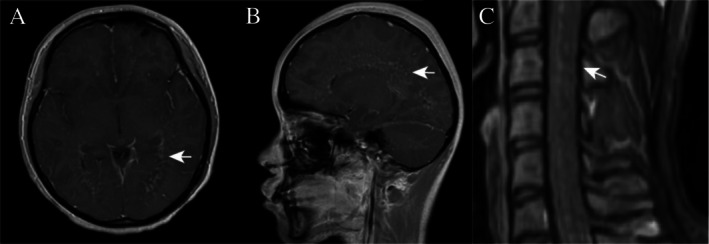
MRI brain w/wo before treatment, post‐contrast. T1 weighted images (A–C) showing patchy perivascular enhancement of the corona radiata area and enhancement in cervical spine.
